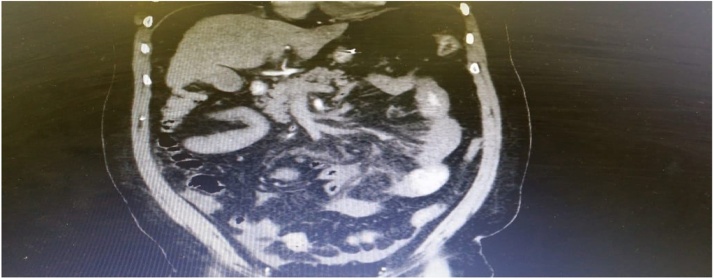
Computed tomography (CT) of the abdomen showed extensive portal, superior mesenteric vein and splenic vein thrombosis.
